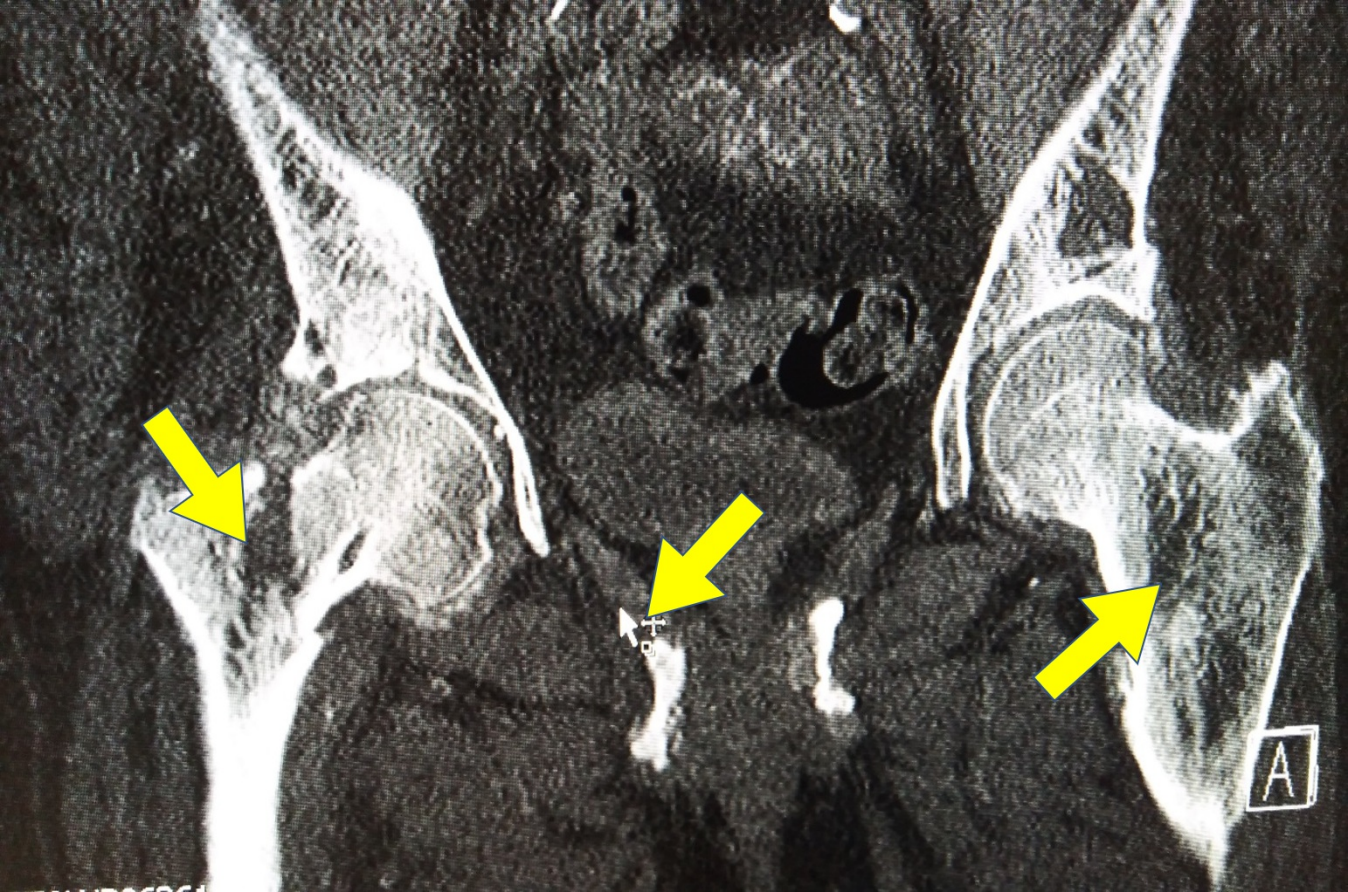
Computed tomography (CT) scan of the pelvis - axial view The fracture is visible with extensive bone resorption not only in the right femur neck but also in the ischium and contralateral femur.
